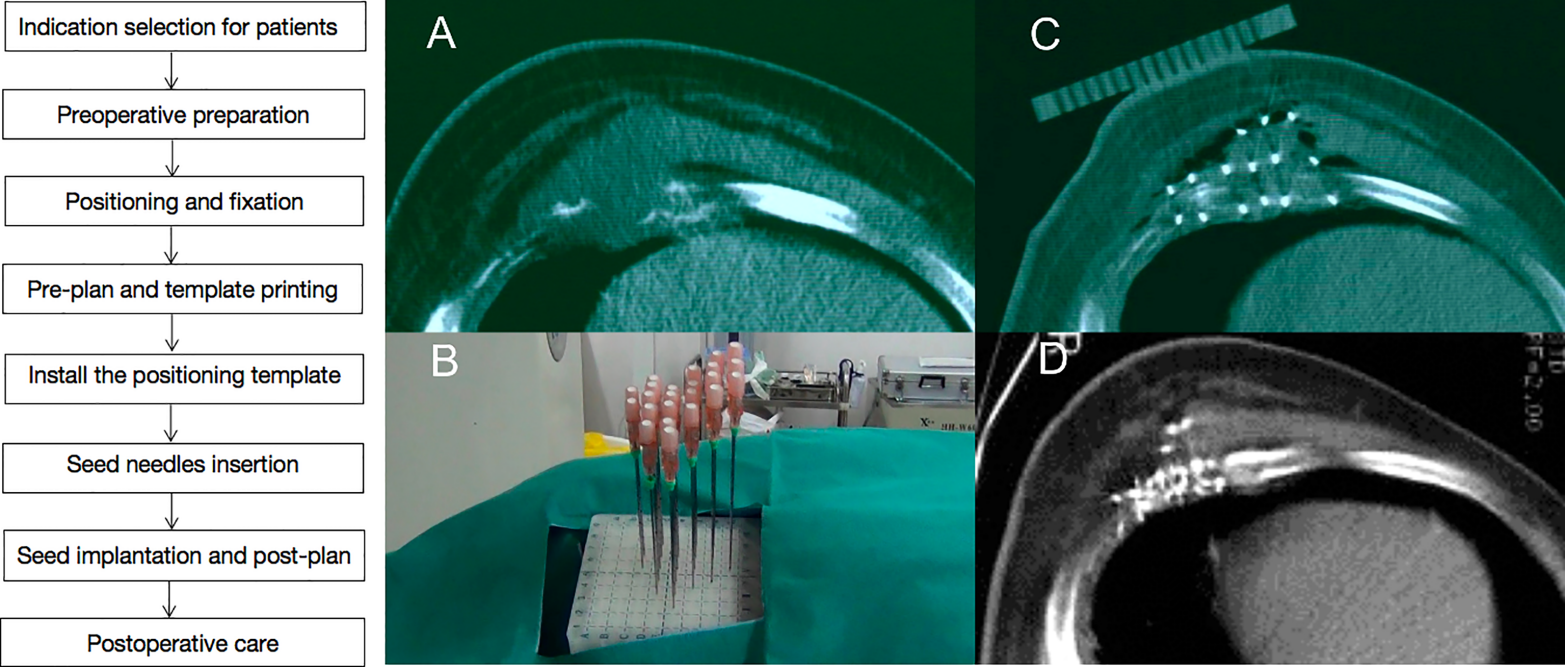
A case of recurrent chest wall cancer (rCWC) following surgery and adjuvant radiotherapy. (A) CT images of rCWC; (B) template-assisted CT-guided puncture the tumor target area; (C)
